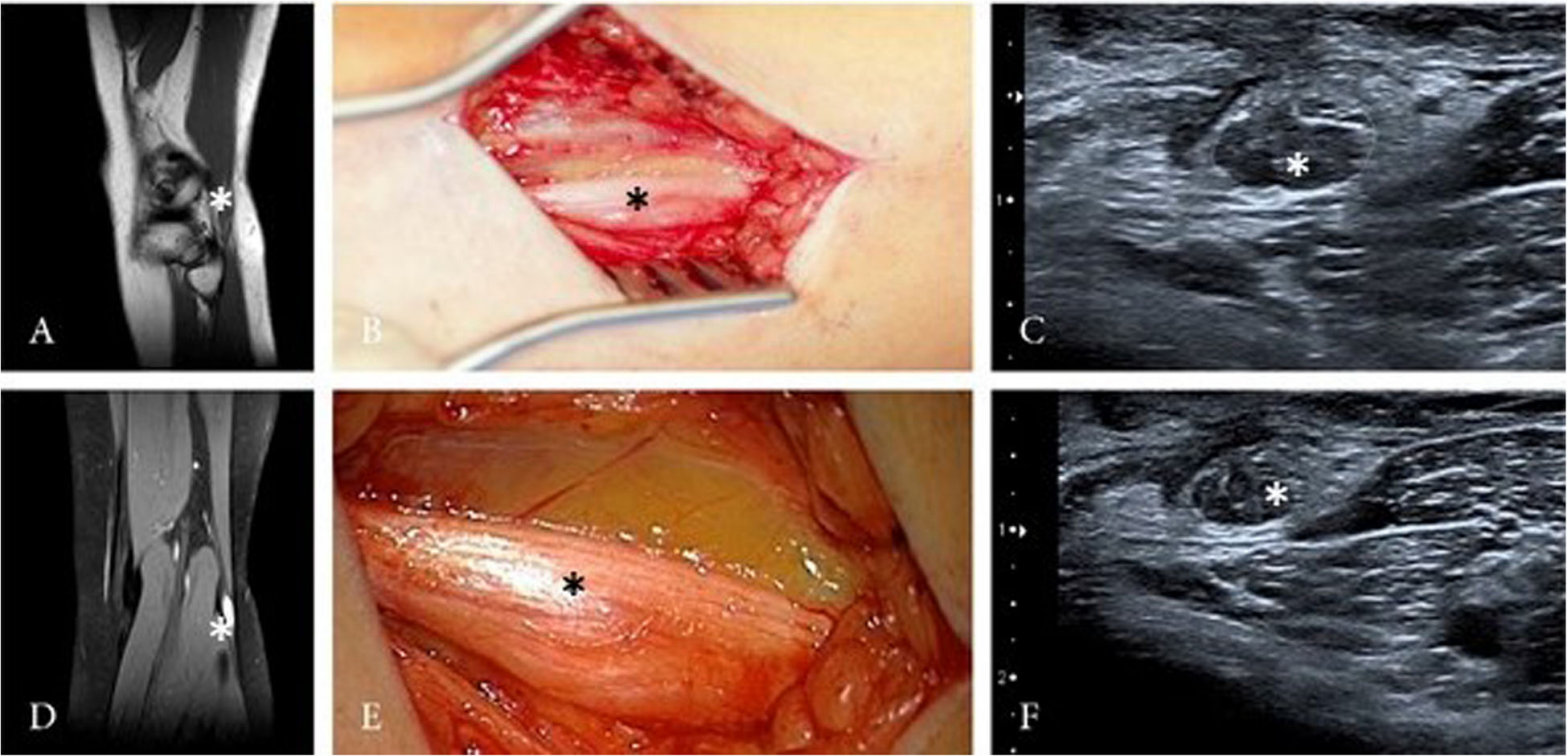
HRNS (c + f) and MRI (a + d) of the left upper limb depicted nerve enlargement (asterisk) with perineurial tissue and still identifiable fascicular structure. Intraoperative view showing the perineurioma (b + e)
